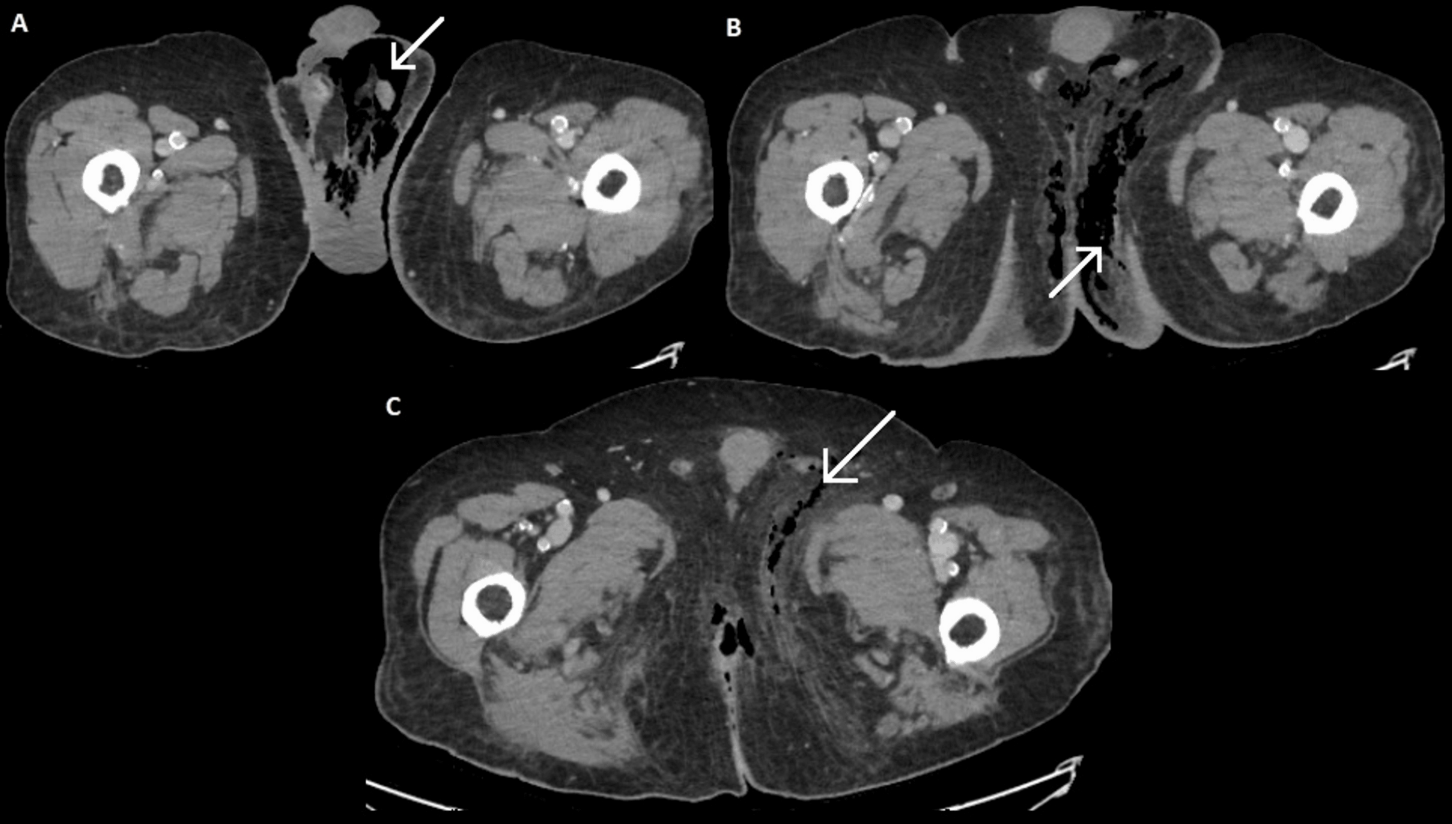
CT scans revealing extensive necrotic involvement of the pelvic floor. CT scan images in portal venous phase showing extensive gas within the scrotum (A) and ischioanal fossae (B), extending superiorly into the left inguinal canal (C).
